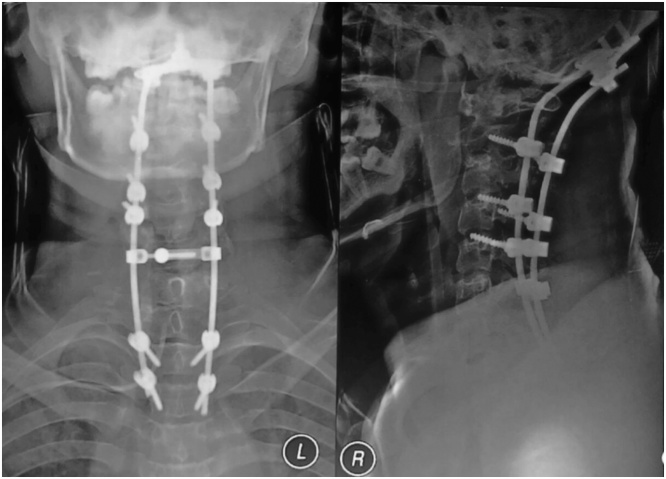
X-ray Cervical AP and Lateral after surgery.
